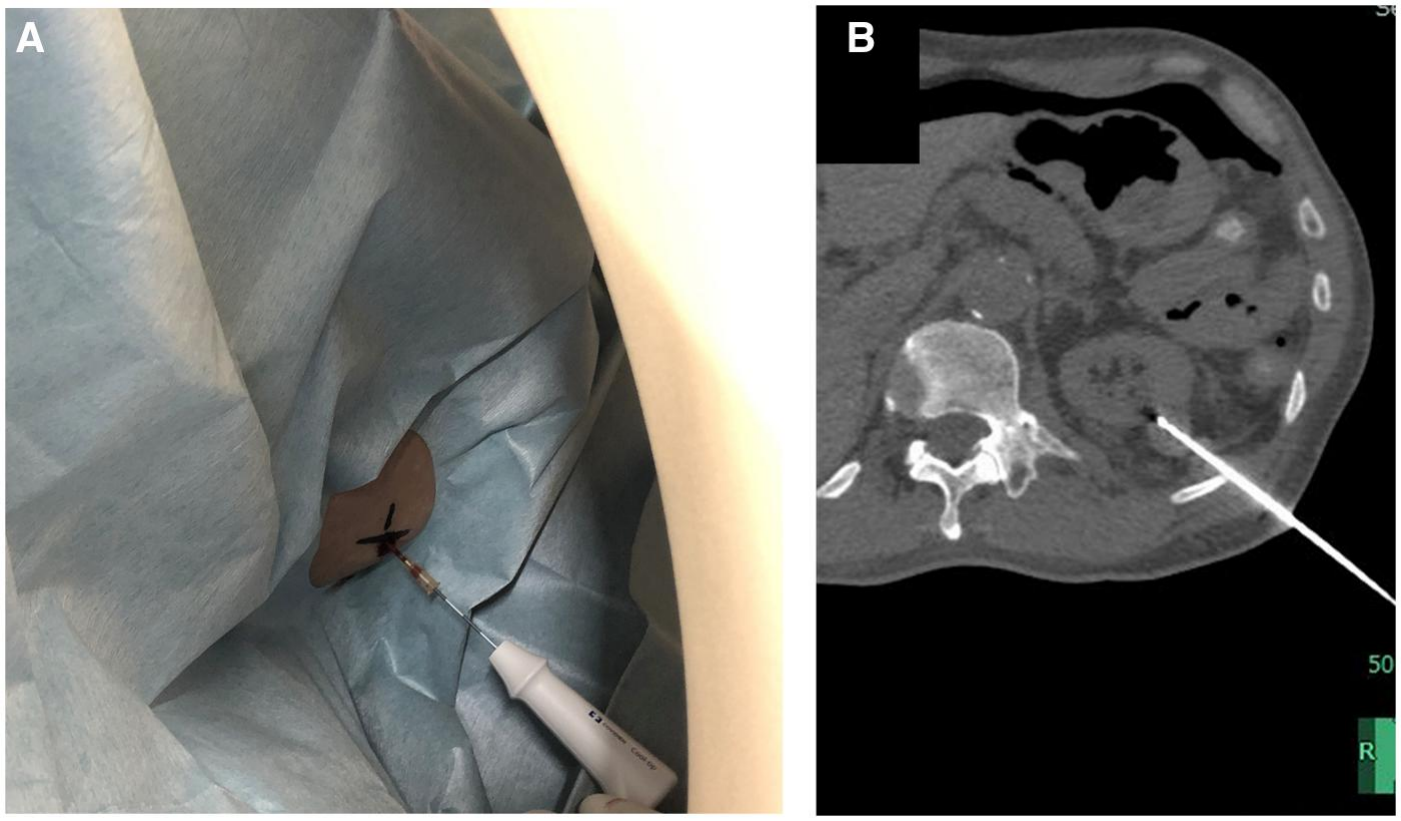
A 61-year-old man undergoing RFA for a right renal tumour. (A) The patient is supine and the RF needle is introduced through the left lateral dorsal aspect. The RF needle does not interfere with the CT gantry or the table. (B) CT image after insertion of the RF needle into the renal tumour using CT fluoroscopic guidance. The polyorefin mat is not visible in normal window setting, but there is space on the patient’s dorsal side.
